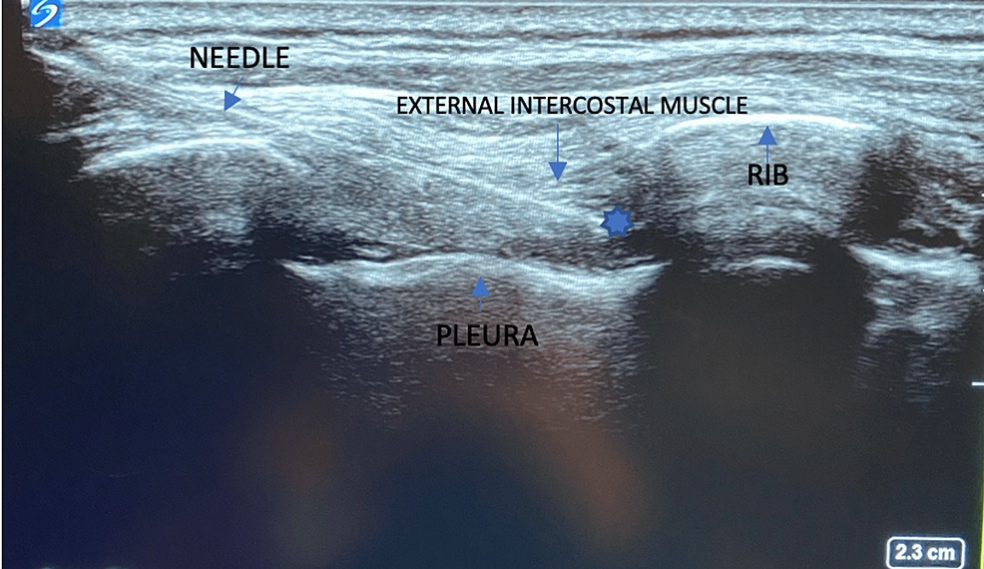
Ultrasound-guided image of thoracic 4 intercostal cryoneurolysis
